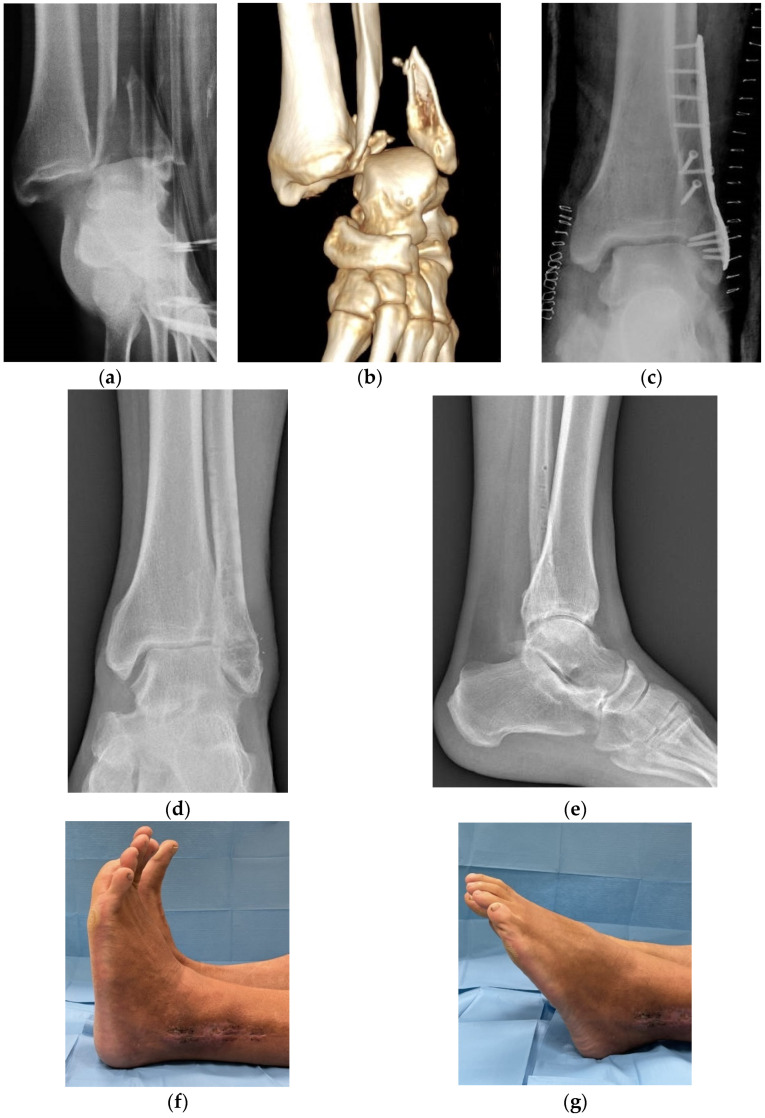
Radiographic examination with a 3D CT scan reconstruction of a fracture-dislocation of the left ankle in a 45 year-old man (a,b) treated by open reduction and internal fixation. The post-operative X-rays showed an anatomic reduction of the lesion with a stable osteosynthesis (c). At follow up, performed 3.8 years later, after hardware removal, AOFAS was 85 points, in spite of a good range of motion of the ankle and the absence of significant radiographic signs of osteoarthritis (Van Dick grade 0) (d–g).
